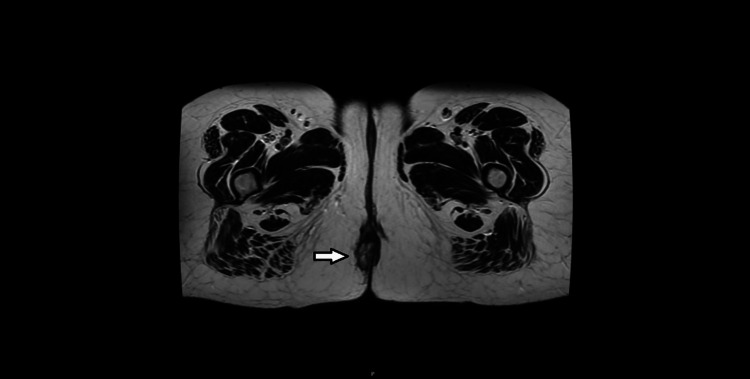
Axial T2-weighted MRI scan of the pelvis with an arrow demonstrating a heterogeneous lobulated lesion in the perianal area
